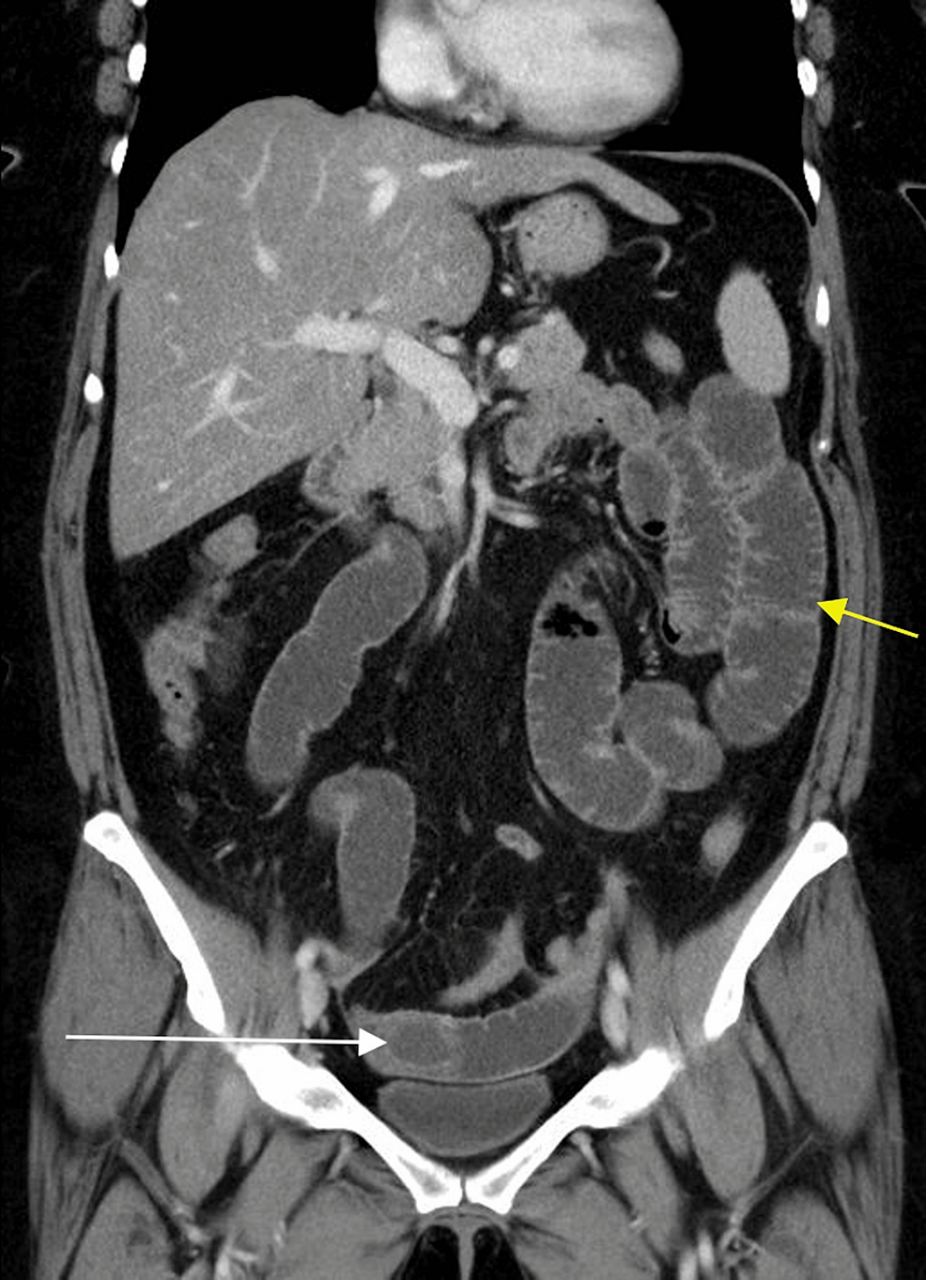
Contrast-enhanced CT of the coronal view demonstrating an obstructing mass in the distal ileum (white arrow) with proximally dilated small bowel (yellow arrow). The mass has the typical appearance of a gallstone.
